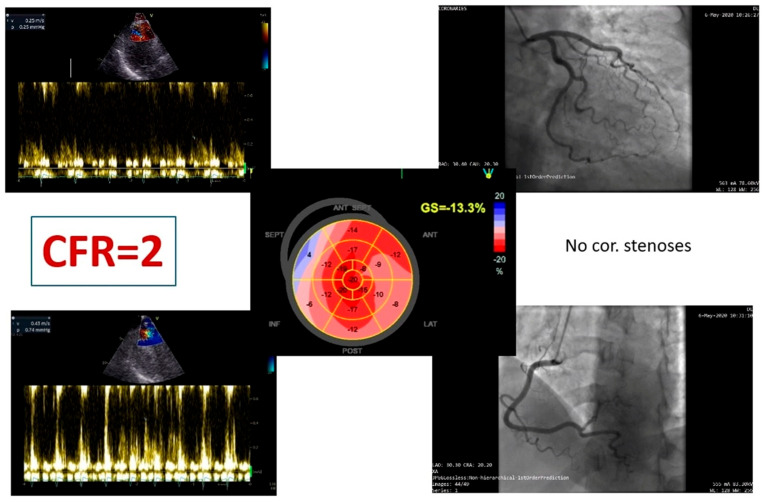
Coronary Microvascular Assessment Using Transthoracic Doppler Echocardiography and Coronary Angiography. (Left panels): Transthoracic Doppler echocardiography of the left anterior descending artery (LAD) at rest (upper-left) and during hyperemia (lower-left), demonstrating diastolic-dominant coronary flow used for calculation of coronary flow reserve (CFR). (Right panels): Coronary angiography showing no obstructive epicardial coronary stenoses. The central polar strain map shows reduced global longitudinal strain (GLS = 13.3%). The combined Doppler recordings yield a CFR = 2, consistent with microvascular dysfunction despite angiographically normal coronary arteries.
